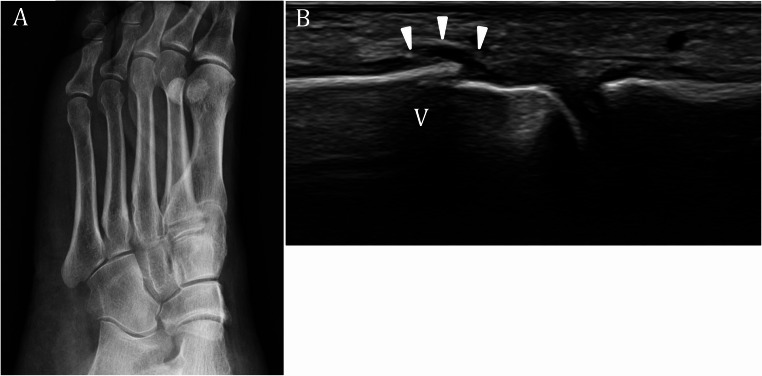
Occult fracture of the fifth metatarsal bone. A. Negative radiographic examination of the foot performed after a trauma. B. On long axis US image, an interruption (arrowheads) of the cortical profile of the fifth metatarsal bone (V) is visible, consistent with a fracture
